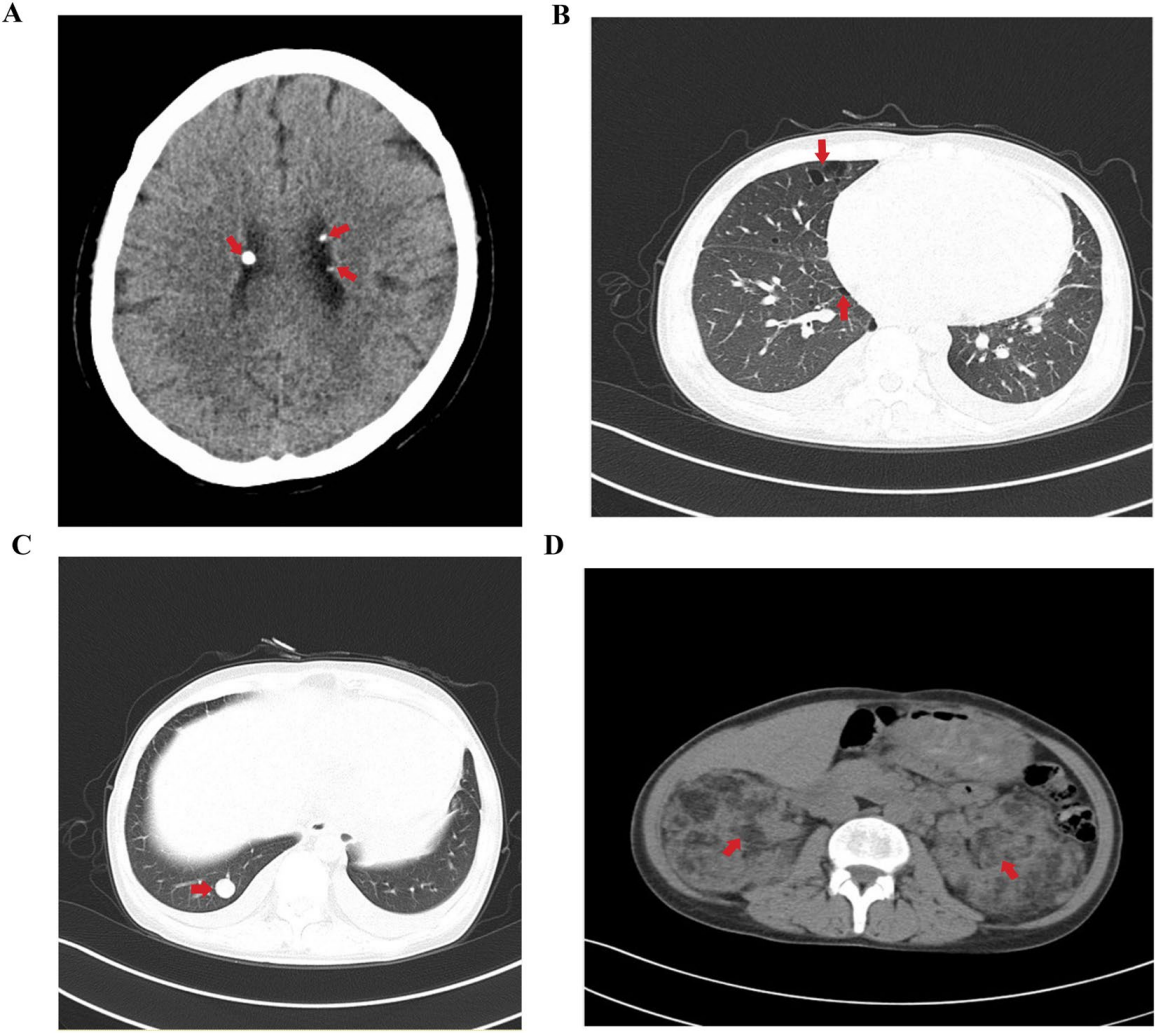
The CT scan of the proband’s head, lung, and abdomen. (A) The head CT scan of the proband. Nodular calcifications in the bilateral ventricle (arrow). (B) The lung HRCT scan of the proband. Multiple bullae in right lung (arrow). (C) The lung HRCT scan of the proband. Pulmonary nodule in right lung (arrow). (D) The abdominal CT scan of the proband. Bilateral renal angiomyolipoma (arrow).
